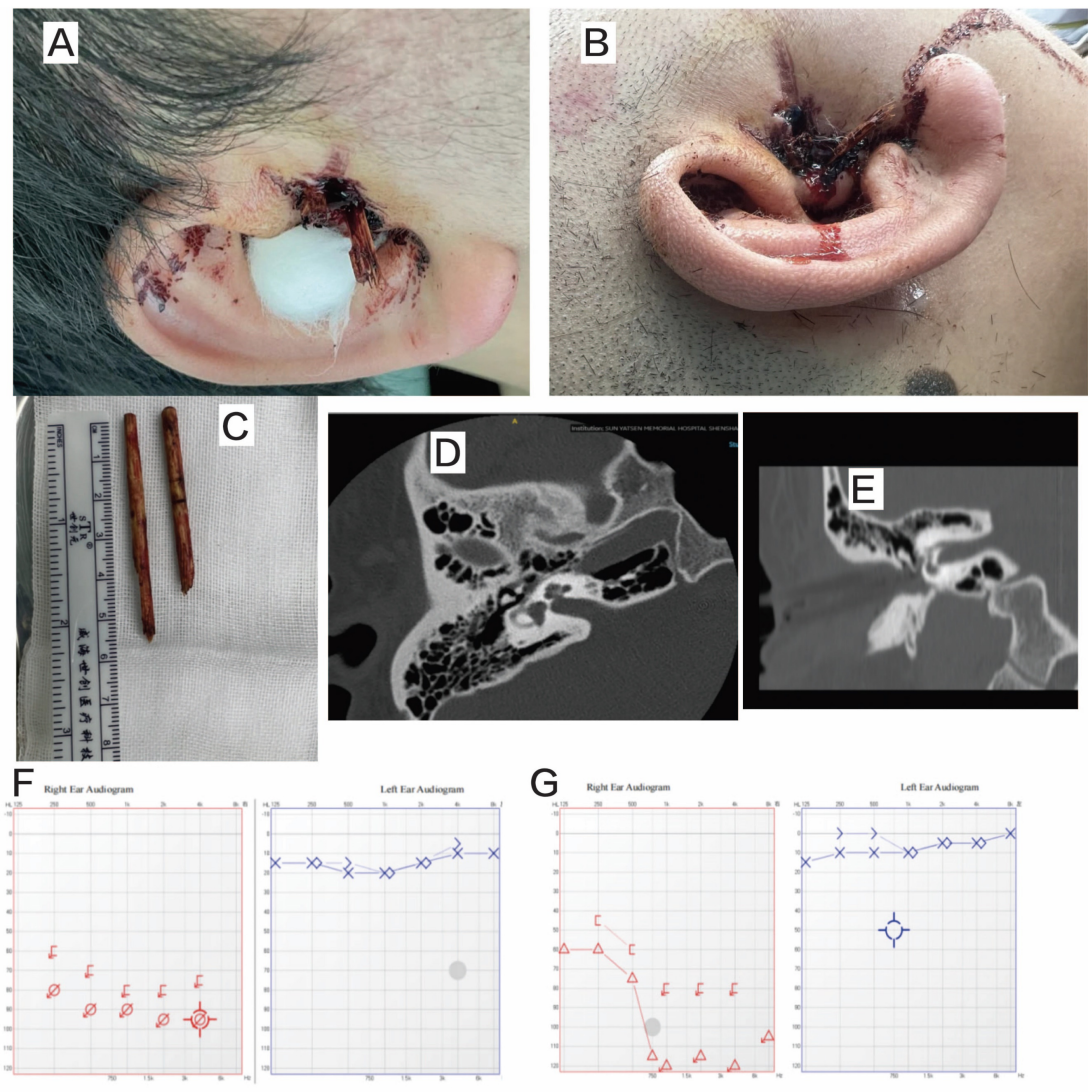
Patient 14. A and B: penetrating trauma to the external auditory canal (bamboo chopsticks). C: the foreign body (bamboo chopsticks). D and E: preoperative HRCT. F: preoperative audiogram. G: postoperative audiogram.
